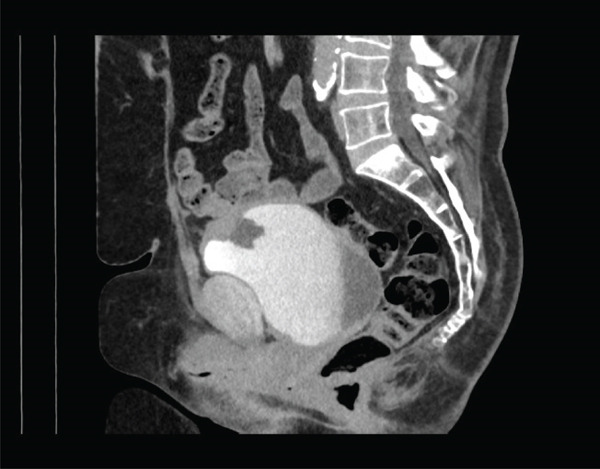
Computed tomography intravenous pyelography study (sagittal view).
